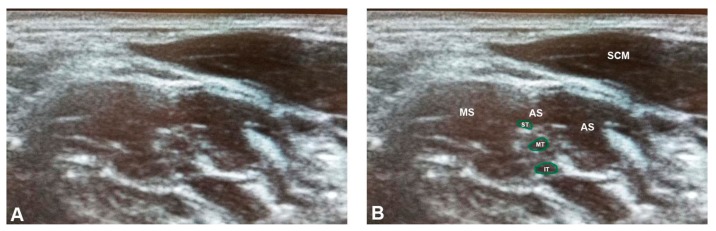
The superior piercing variant, the most common brachial plexus variant, identified using ultrasonography in the present study: (A) unlabeled; and (B) labeled. Note that the superior trunk is not separated from the anterior scalene in this condition, visible as a lack of hyperechoic fascia. AS = anterior scalene; IT = inferior trunk; MS = middle scalene; MT = middle trunk; SCM = sternocleidomastoid; ST = superior trunk. The green outlines demarcate the trunks of the brachial plexus.
